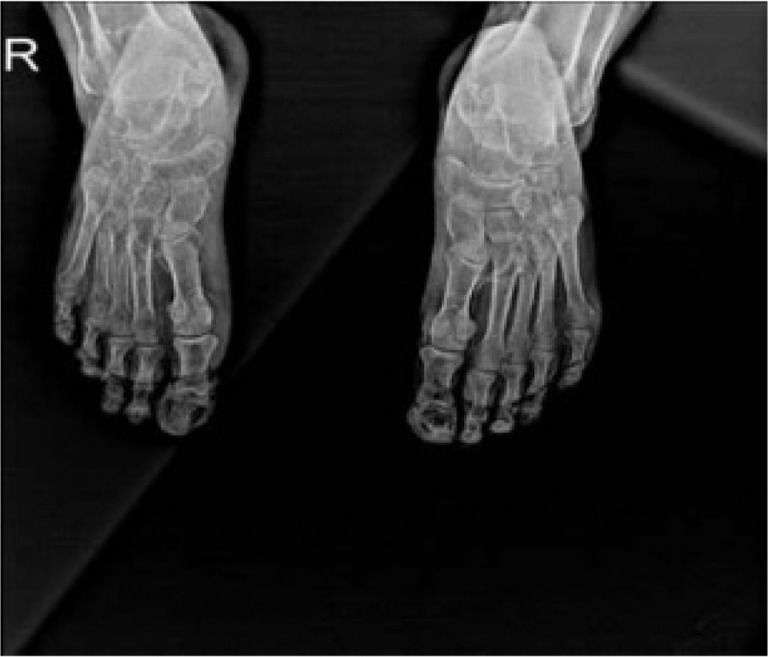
Feet X-ray showing calcifications (showed with arrows) of our second case.
